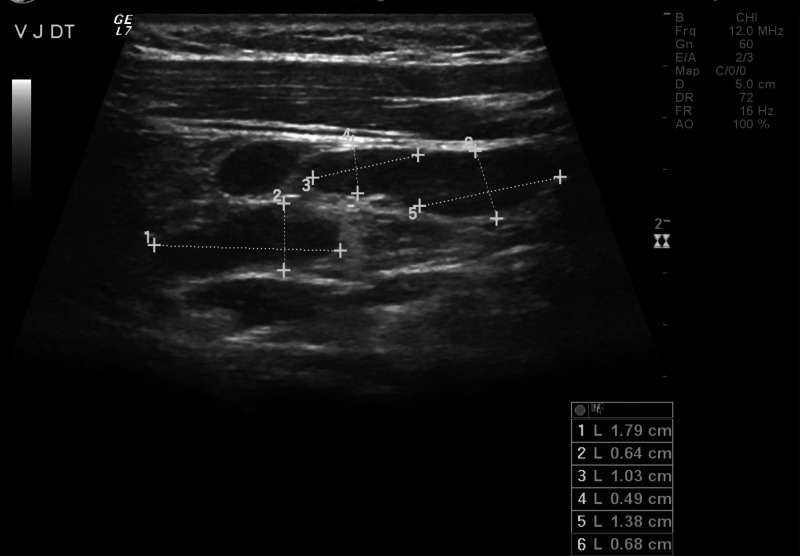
Cervical ultrasound of the patient The cervical ultrasound showed bilateral multiple lymph node conglomerates (indicated by the dotted lines with the respective dimensions on the right lower corner)
